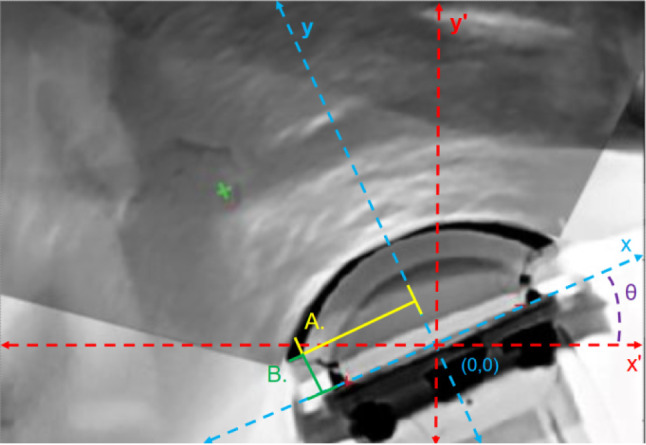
Axes and measurements for registration of ultrasound images to videofluoroscopic imaging. Axes (*x*,*y*) and (*x*’,*y*’) respectively correspond to Cartesian coordinate systems for B-mode ultrasound and videofluoroscopy image sequences, with the common origin (0,0) defined as the position of a tracked reference point within the transducer at the initial frame. The rotational angle between axes of these Cartesian coordinate systems is θ. (A) Line segment used to measure horizontal (*x*) distance from tracked corner of B-mode images to the origin. (B) Corresponding line segment used to measure vertical (*y*) distance from tracked corner of B-mode images to the origin
